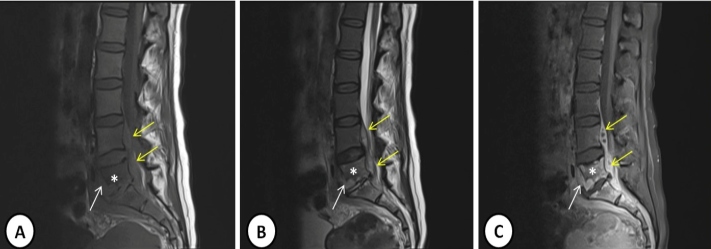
Lumbar magnetic resonance imaging consistent with an abscess at the L4-S2 vertebral level, appearing hypointense in T1 -weighted sections (A), hyperintense in T2-weighted images (B), and with intense contrast enhancement in post-contrast sections (C) (yellow arrows). Pathological signal changes compatible with spondylodiscitis exhibiting extension to the anterior epidural fatty planes are visible in the corners of the L5 vertebra (*) and S1 corpus and L5-S1 intervertebral disc intensity (white arrow).
